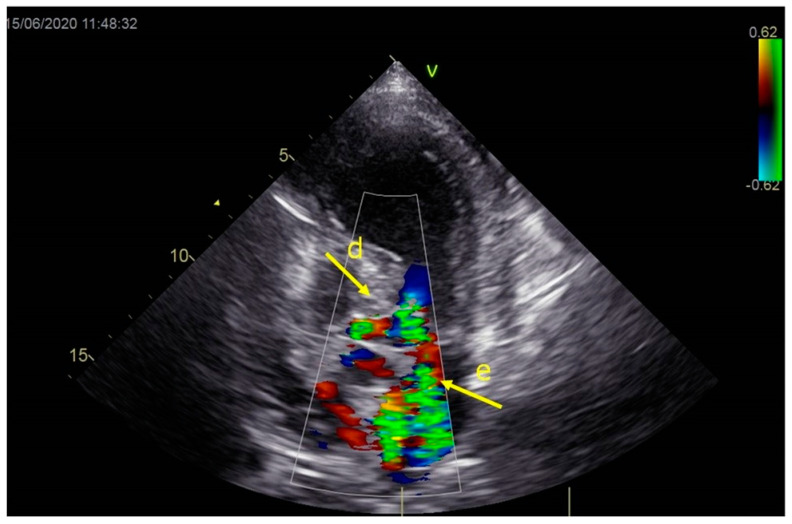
Echocardiography registered moderate mitral regurgitation (d—LVOT, e—mitral regurgitation).
